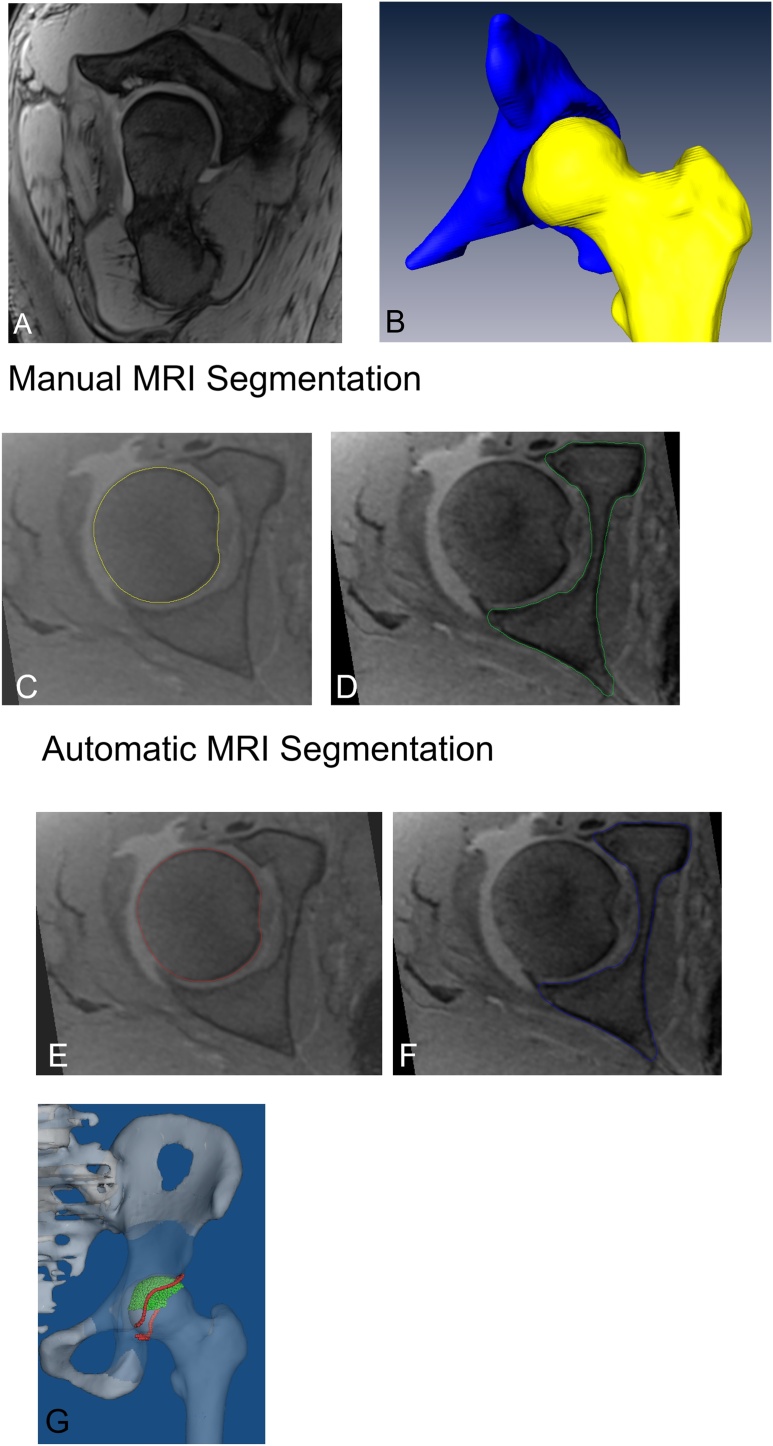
A-G The MRI image (A), an MRI-based 3D model (B) and comparison between manual (C and D) and automatic MRI-based 3D model (E and F) of the femur (C and E) and of the acetabulum (D and F) are shown. Calculation of femoral head coverage (G) is shown.
